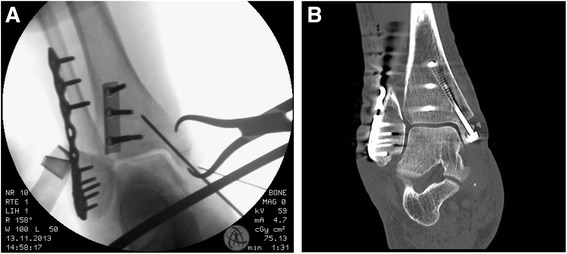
Intraoperative x-ray; a Mortise view with an antiglide-plate for the posterior malleolus, locking plate for the fracture of the lateral malleolus and additional temporary k-wire fixation of the medial malleolus. b postoperative CT-scan (coronar view) with an antiglide-plate for the posterior malleolus, locking plate for the fracture of the lateral malleolus and lag screw fixation of the medial malleolus
